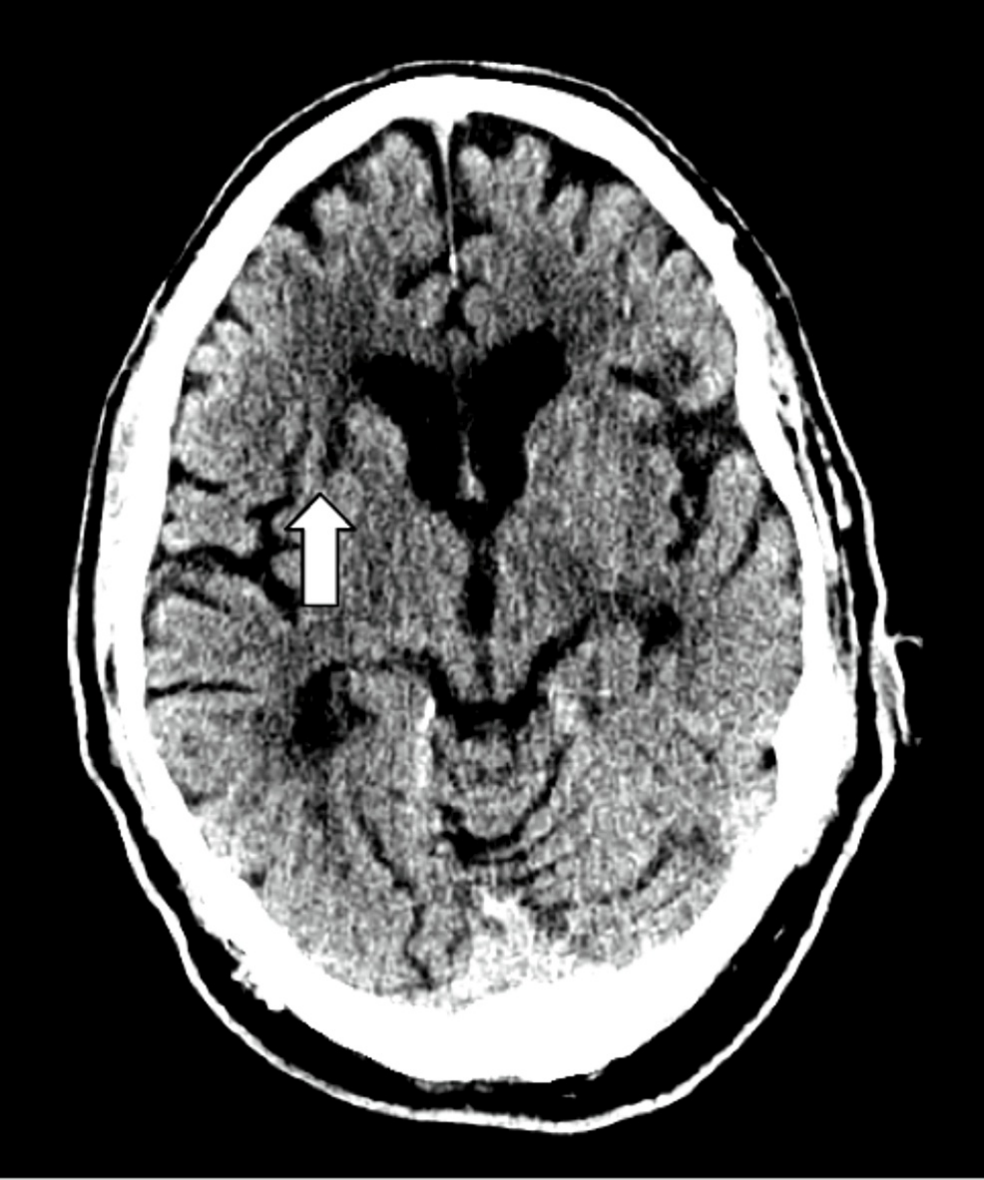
Standard view of CT brain without contrast demonstrating right lacunar infarct within basal ganglia as indicated by the white arrow
